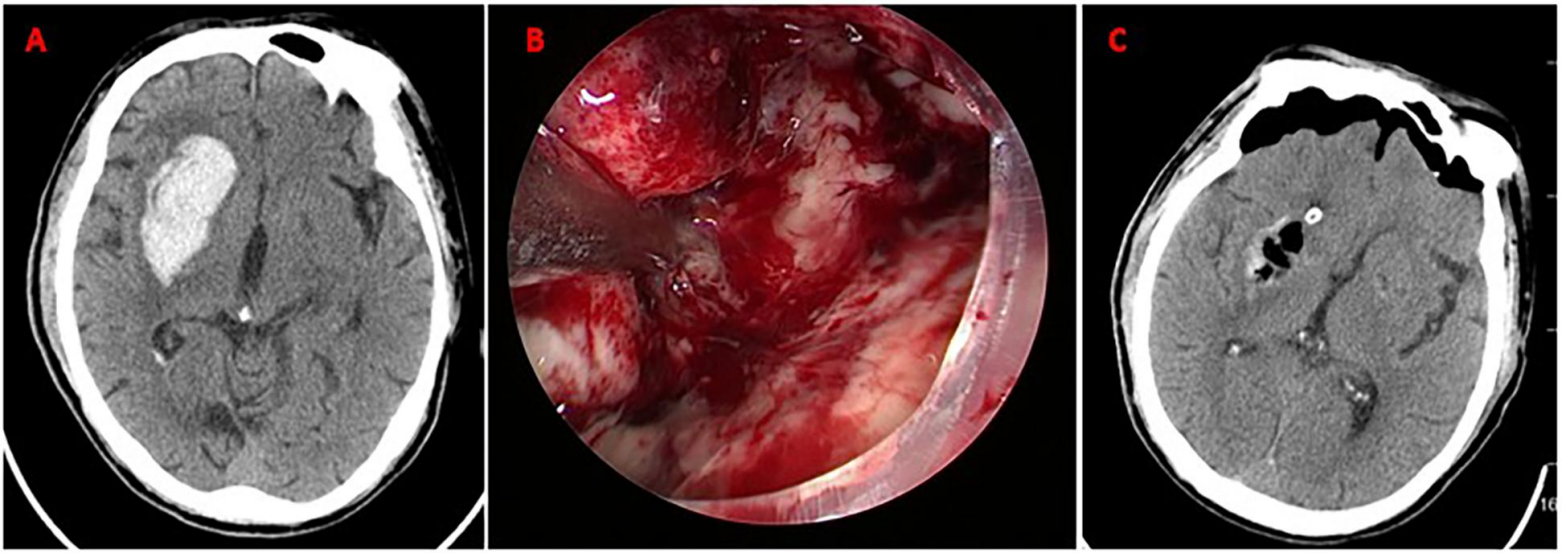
Neuroendoscopic evacuation of right basal ganglia hematoma. (A) Axial CT demonstrates acute hypertensive hemorrhage (volume: 35 mL) in the right basal ganglia; (B) Intraoperative neuroendoscopic view during hematoma evacuation via a frontal approach. The hematoma cavity is visualized; (C) Postoperative axial CT scan confirming near-total hematoma evacuation (residual volume <2 mL). No new ischemic lesions or rebleeding are observed. Mild perihematomal edema is present.
